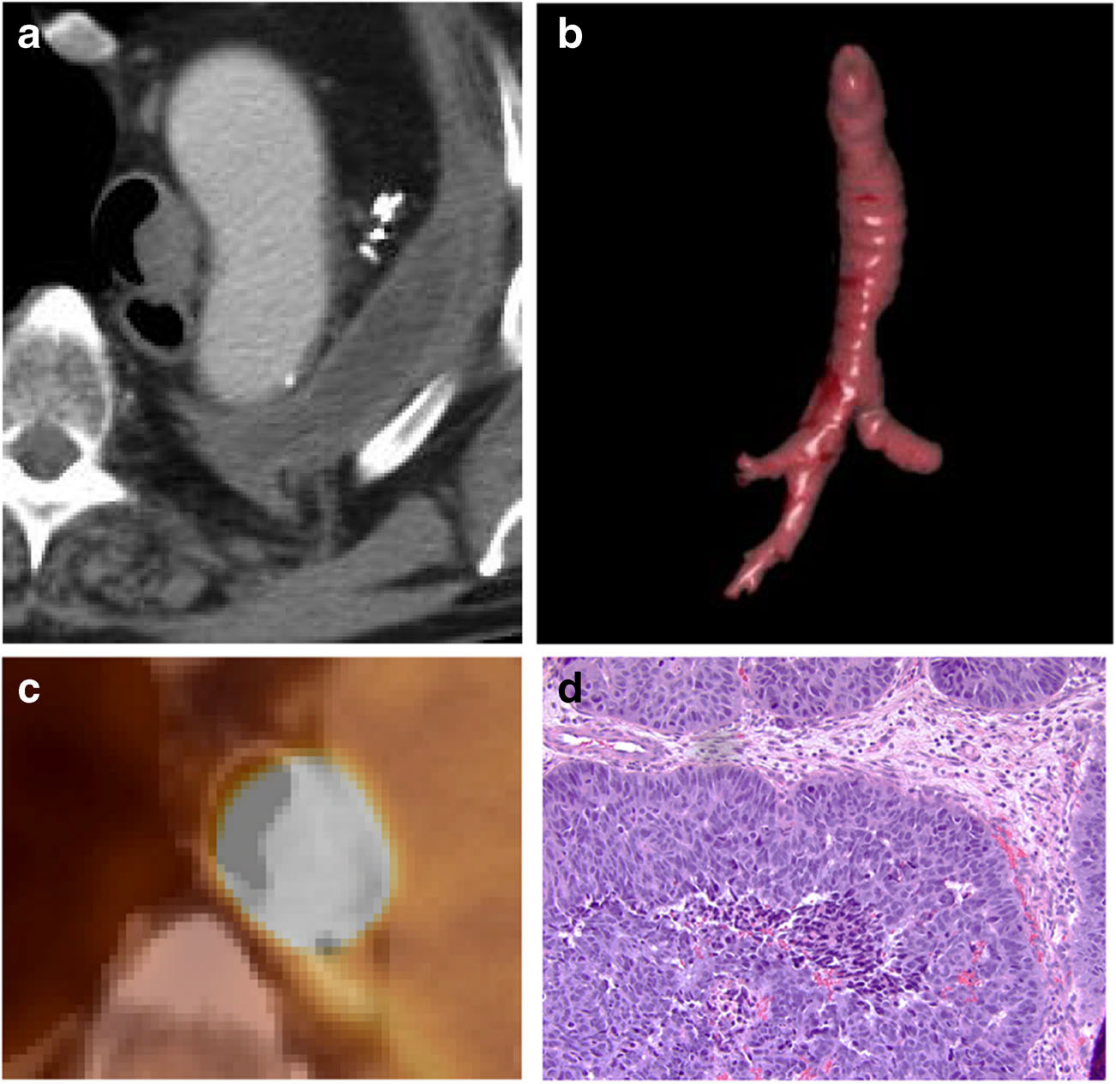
Sixty-six-year-old man with past medical history of squamous cell lung carcinoma. a Axial CT demonstrates left posterolateral tracheal wall mass. b 3D VR image demonstrates focal narrowing of the distal trachea. c PET/CT demonstrates high FDG avidity. d Pathology reveals invasive squamous cell carcinoma with basaloid morphology
